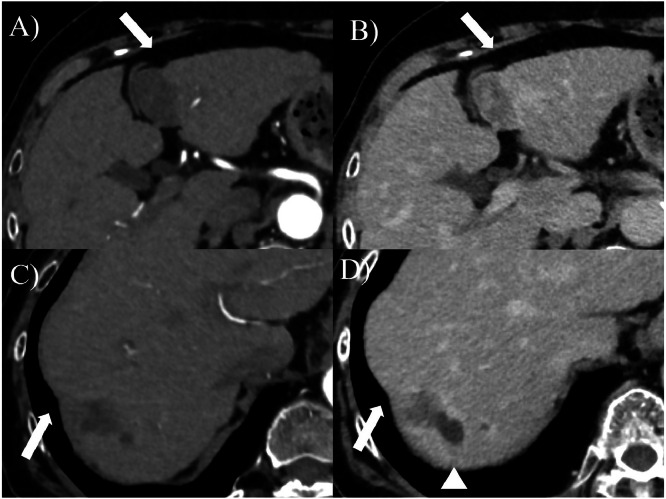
Dynamic abdominal computed tomography findings (2024+23 years). Atrophic masses measuring 29×24 mm in liver S2/3 (A, B) and 18×17 mm in liver S8 (C, D) are observed. In the arterial phase, peripheral nodular enhancement disappeared (A, C). In the delayed phases, only slight contrast enhancement is observed in the center of the tumor (B, D). A concave deformation of the liver surface is observed (arrow). The cyst near S8 has expanded (arrowhead).
